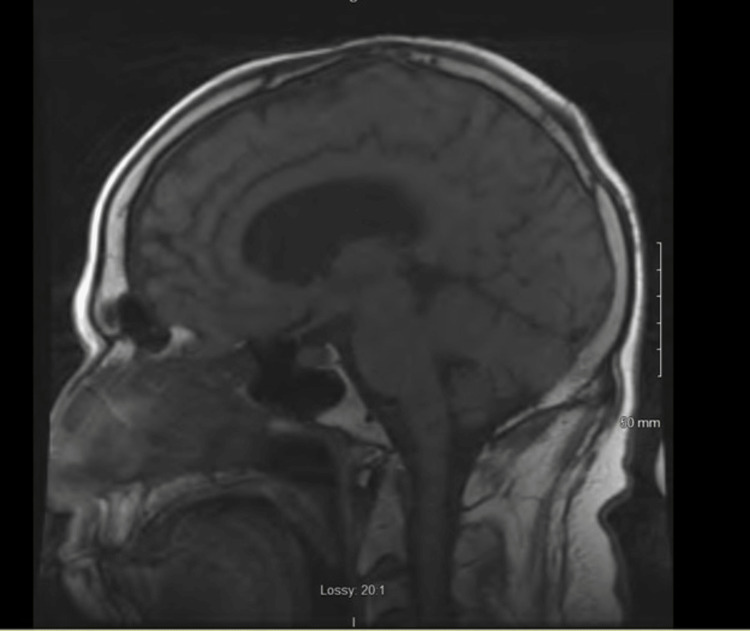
Sagittal MRI showing mild anterior pituitary enlargement, suggestive of possible anterior hypophysitis
